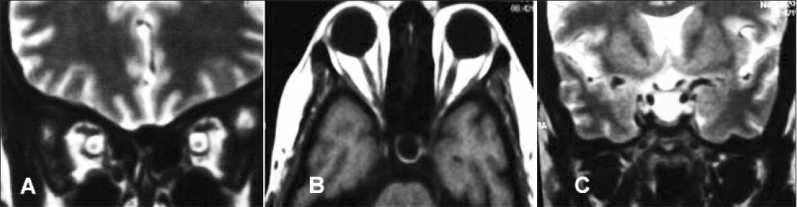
MRI images coronal section through brain and orbit showing widening of the perioptic space (A) and empty sella in axial section T1 sequence (B) coronal section T2 sequence (C)
